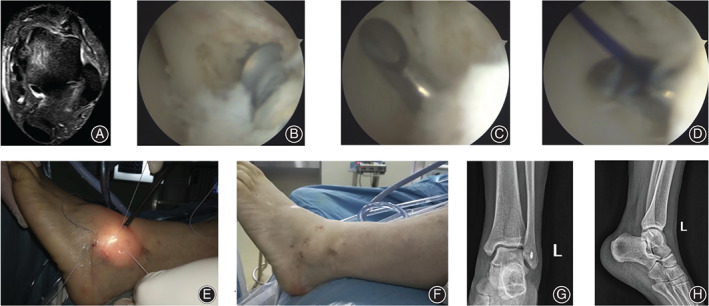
A 45‐year‐old male patient with chronic lateral ankle instability of the left side for 12 months. (A) The preoperative MRI showed the interruption of the ATFL. (B) The intra‐operative view under the arthroscope showed the ATFL interruption, a suture anchor was fixed into the footprint of the distal fibular. (C) A suture passer was used to pass through the ATFL. (D) The PDS II was passed through the suture passer and guided the suture arm to pass through ATFL and the inferior extensor retinaculum. (E) The arms of the suture anchor were knotted by free‐edge suture fashion. (F) The surgical approaches view after the procedure. (G) The postoperative anterior–posterior X‐ray film of the involved ankle. (H) The lateral X‐ray film after the surgery.
